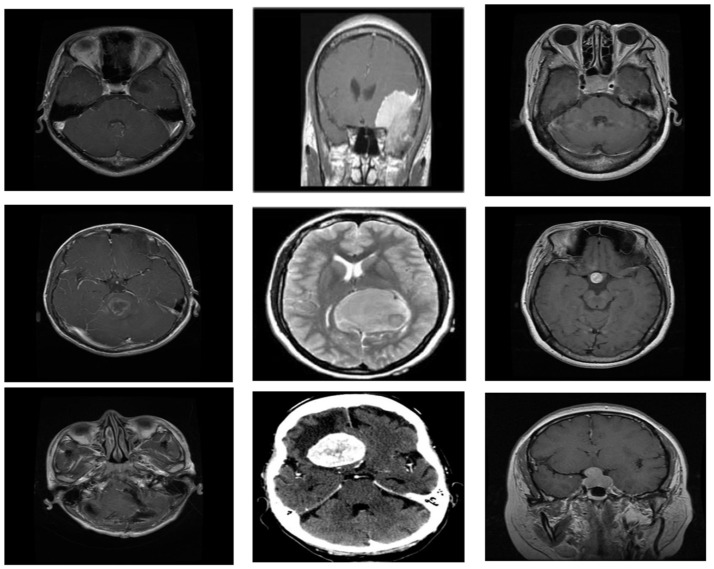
MRI image classes i.e., Glioma (left), Meningioma (Middle), and Pituitary (Right).
